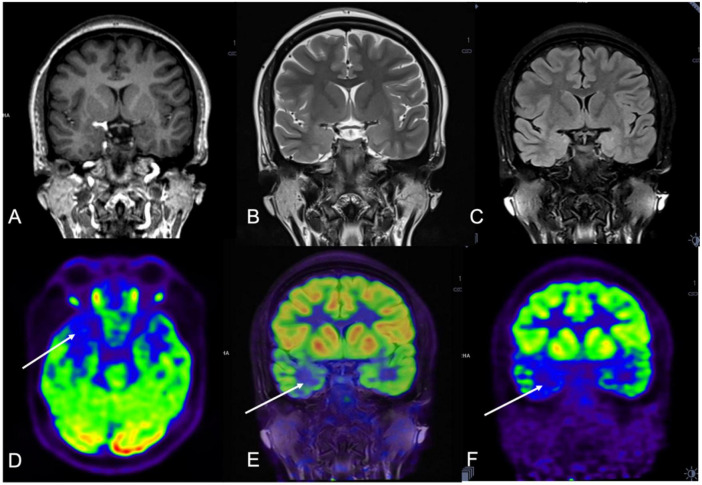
A 21-year-old woman (patient #11) with refractory epilepsy for 1 year. Magnetic resonance (MR) imaging coronal T1 (A), coronal T2 (B), and coronal Flair (C) were negative. Positron emission tomography (PET) (D,F) and hybrid PET/MRI (E) showed focal hypometabolic area in the right temporal lobe. Combined with semiology and stereoelectroencephalography (SEEG) results, the right mesial temporal lobe was hypothesized to be the seizure onset zone (SOZ) and stereoelectroencephalography-guided radiofrequency thermo coagulation (SEEG-guided RFTC) was performed. And the patient was seizure-free at 30-month follow-up.
